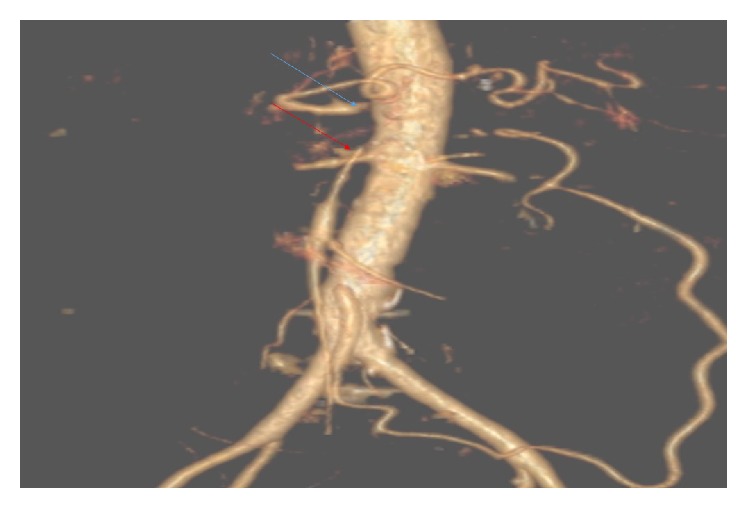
Computed tomography (CT) angiogram shows stenosis of the SMA and celiac trunk origins.
